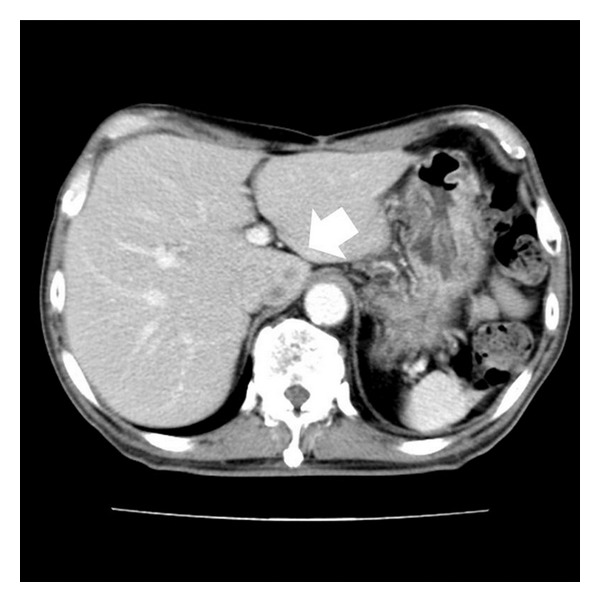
Enhanced computed tomography (CT) of the abdomen. The tumor was detected in the Spiegel of the liver on enhanced CT of the abdomen (arrow).
